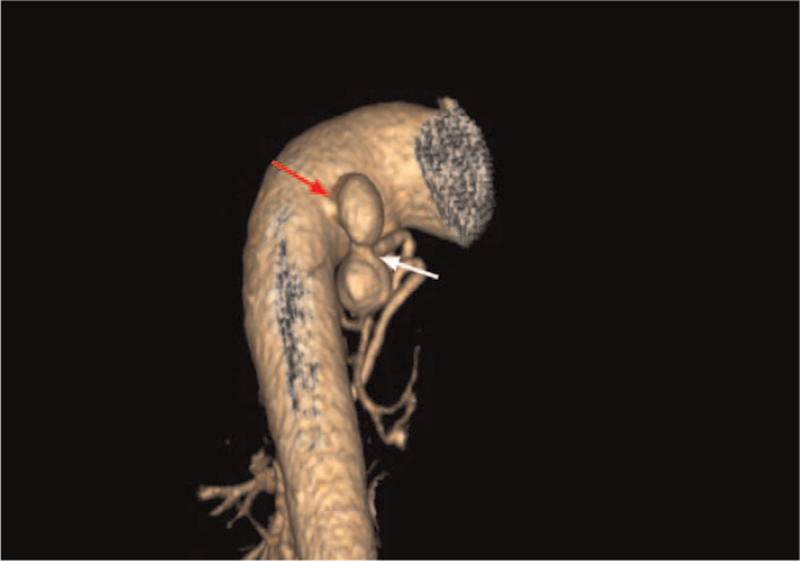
Three-dimensional CT reconstruction of bronchial artery aneurysms. These 2 aneurysms were found in tandem on the same bronchial artery. The first aneurysm (red arrow) originated directly from the aorta and had a narrow neck between itself and the second aneurysm (white arrow).
